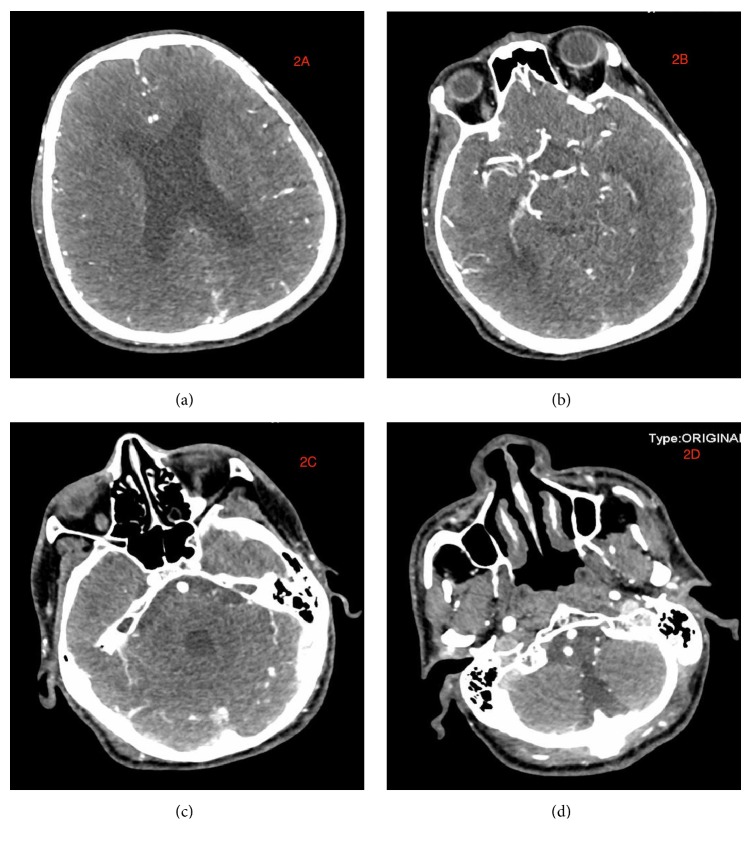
(a)-(d) are CT-scan images indicating no intracranial abnormities after the injury.
